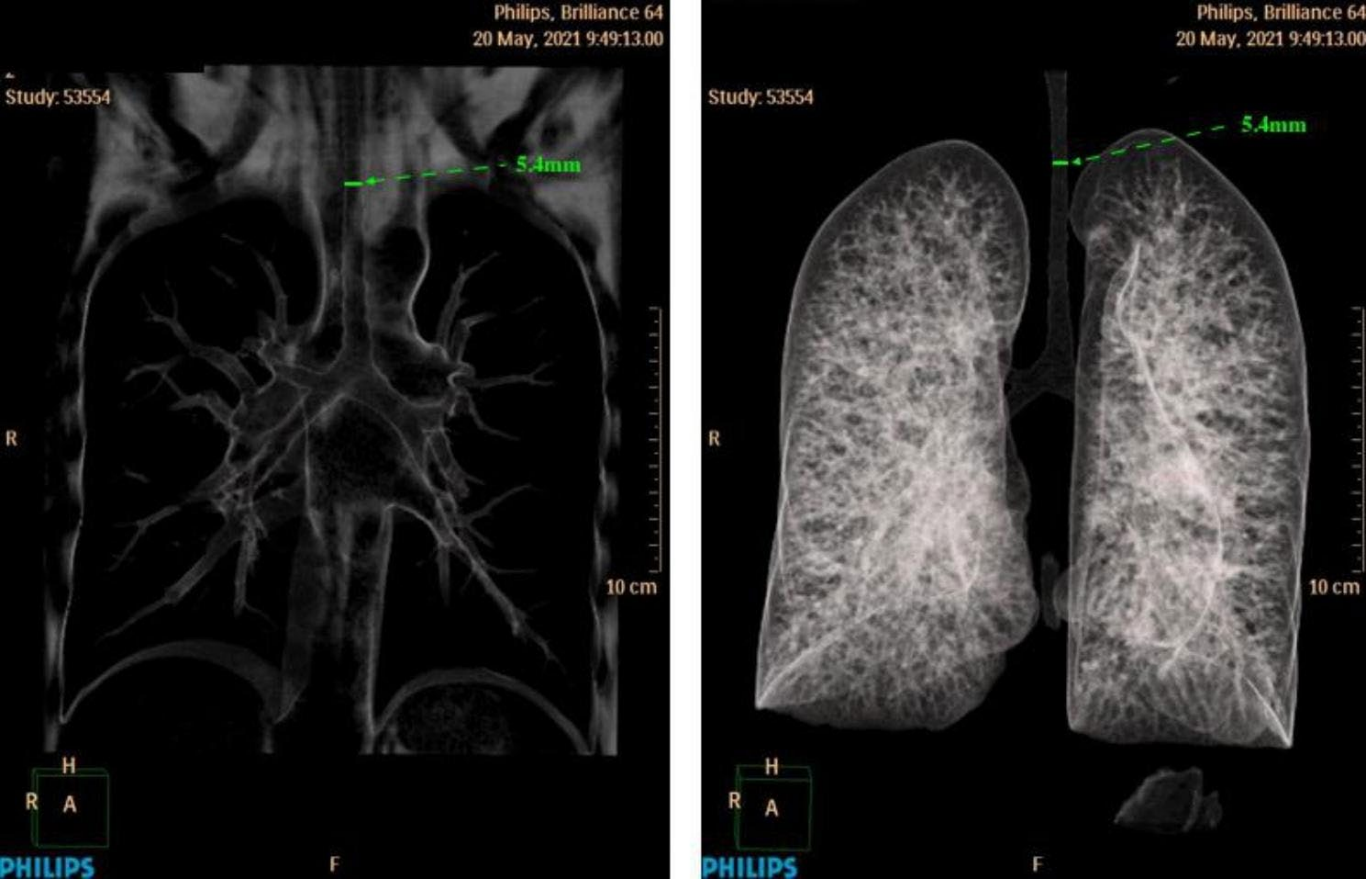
Three-dimensional image reconstruction of chest CT. The minimum diameter of the trachea was only 5.4 mm
